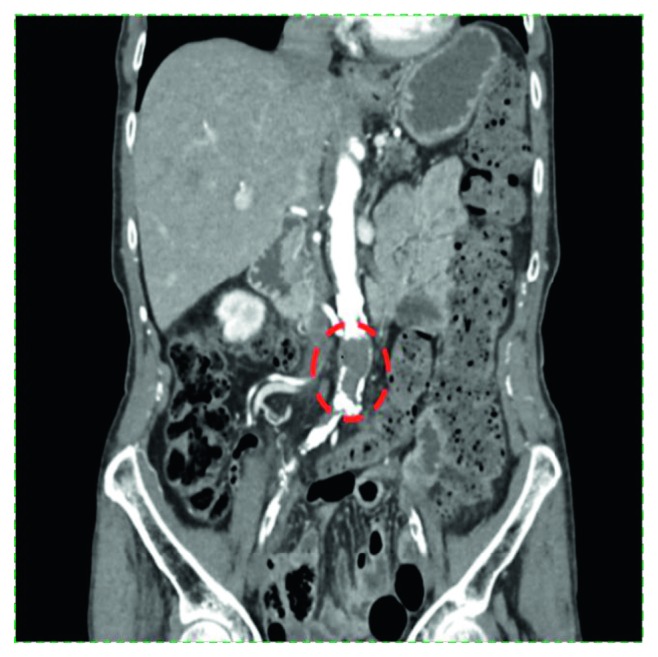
Computed tomography (CT) abdomen and pelvis demonstrating an occlusive thrombus in the abdominal aorta distal to the mesenteric artery. There is thrombus extension into the common iliac arteries with reconstitution of flow distally via collaterals.
